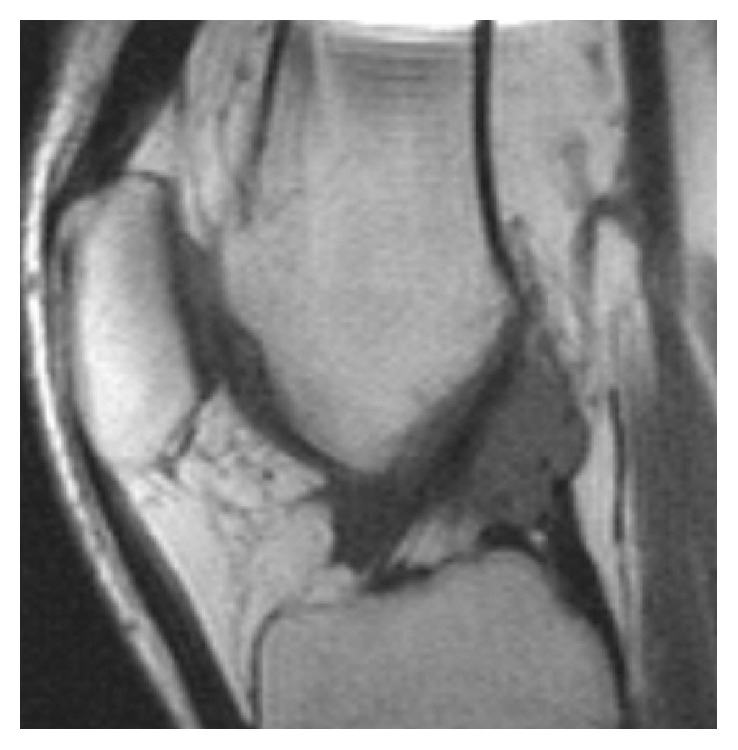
34-year-old man with a small sarcoma at the cruciate ligaments (case #S6). Sagittal T1-weighted MR image.
